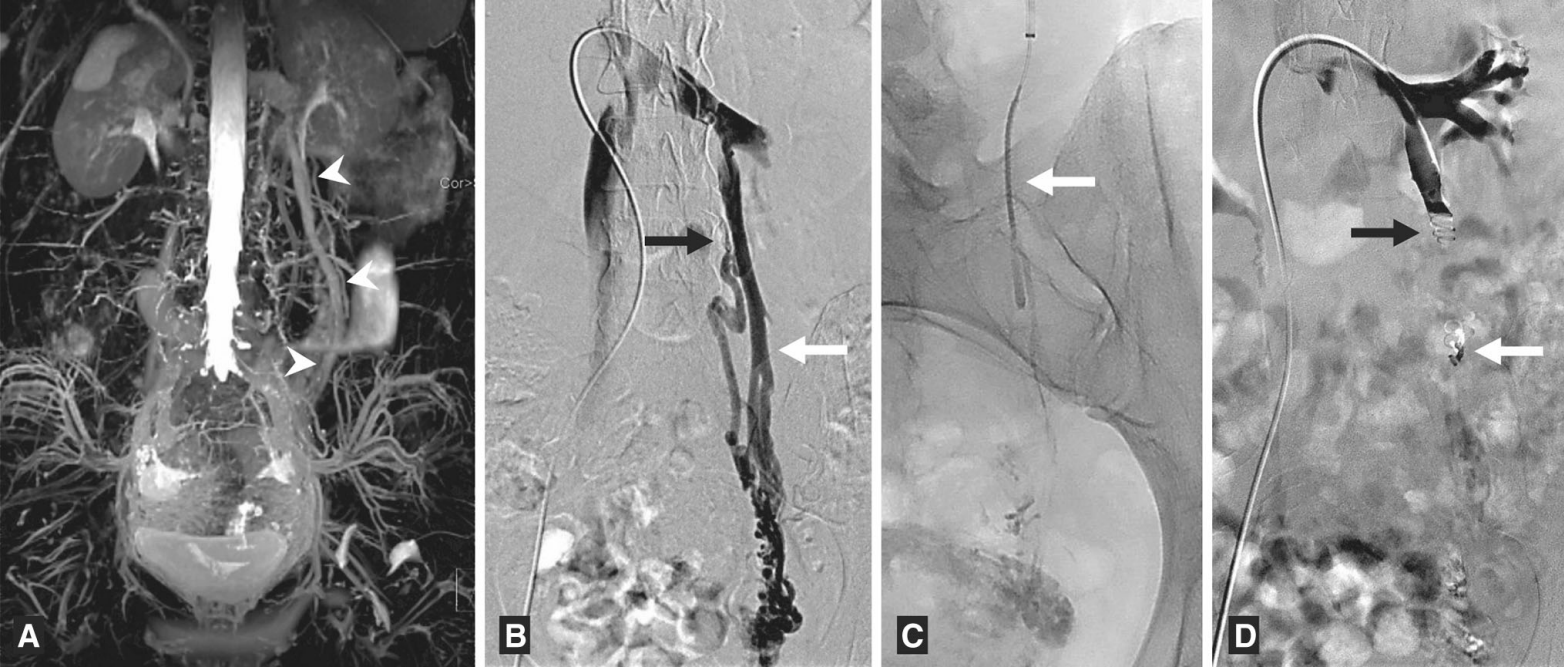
The 22-year-old female with PCS. A The MR appearance of the dilated left ovarian vein (*arrowheads*)—FSE T2-weighted sequence with fat-sat (MIP reconstruction). B Initial venography, showing a complex confluence of dilated ovarian vein. C The first occlusion device on position—right over the lower, major veins confluence (*white arrow*). D Check venography, after placement of the second occluder, implanted over the highest venous confluence (*black arrow*)
